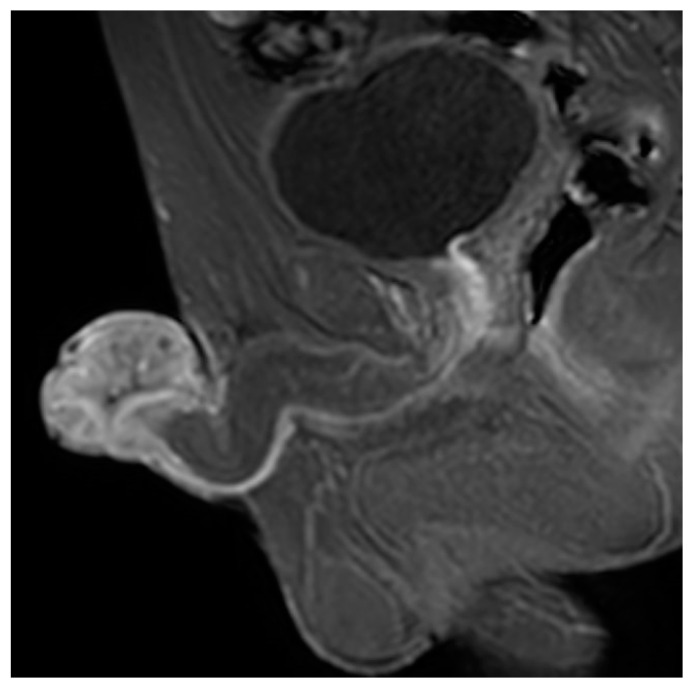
Magnetic resonance imaging (MRI) pelvis showing lobulated T2 (transverse relaxation time) intermediate mass at the level of the glans penis.
